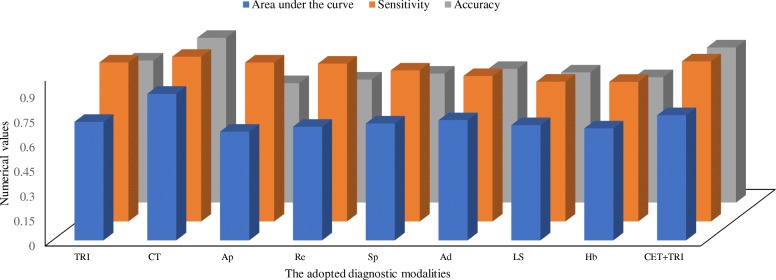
The analogy of diagnostic modalities for decision making of surgeries of the Bankart and bony Bankart lesions. Accuracy and sensitivity of Computed Tomography were considered as 1. One-way repeated-measures ANOVA following Dunnett Multiple comparisons tests was used for statistical analysis. A *p*-value < 0.01 and *q*-value > 4.148 were considered significant. TRI: The traditional radiological images, CT: The Computed Tomography, Ap: Apprehension test, Re: Relocation test, Sp: Surprise test, Ad: Anterior drawer test, LS: Load and shift test, Hb: Hyperabduction test, CET + TRI: Clinical examination tests combining the traditional radiological images
